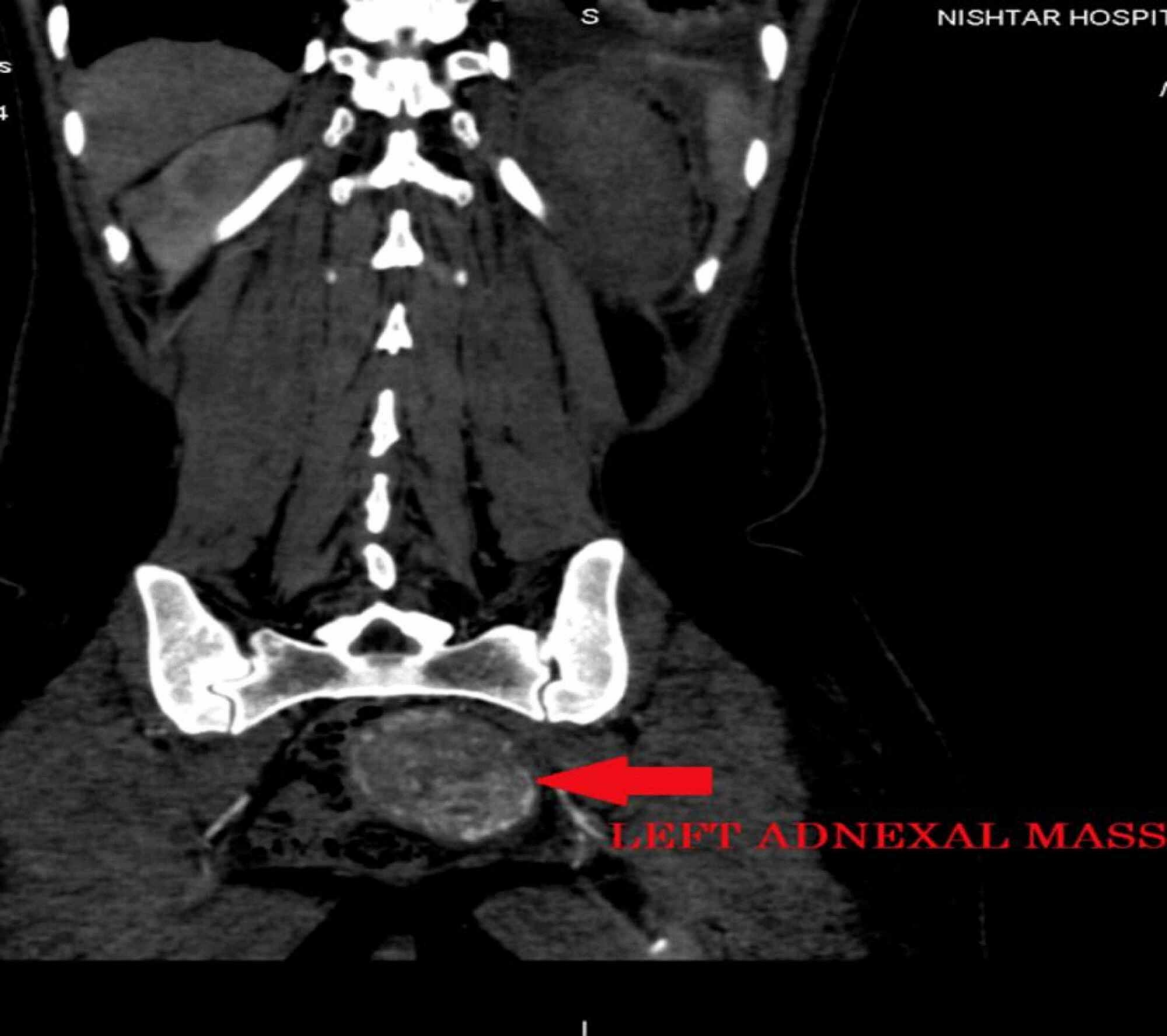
Coronal reformat CT showing an enhancing left adnexal mass CT: computed tomography
